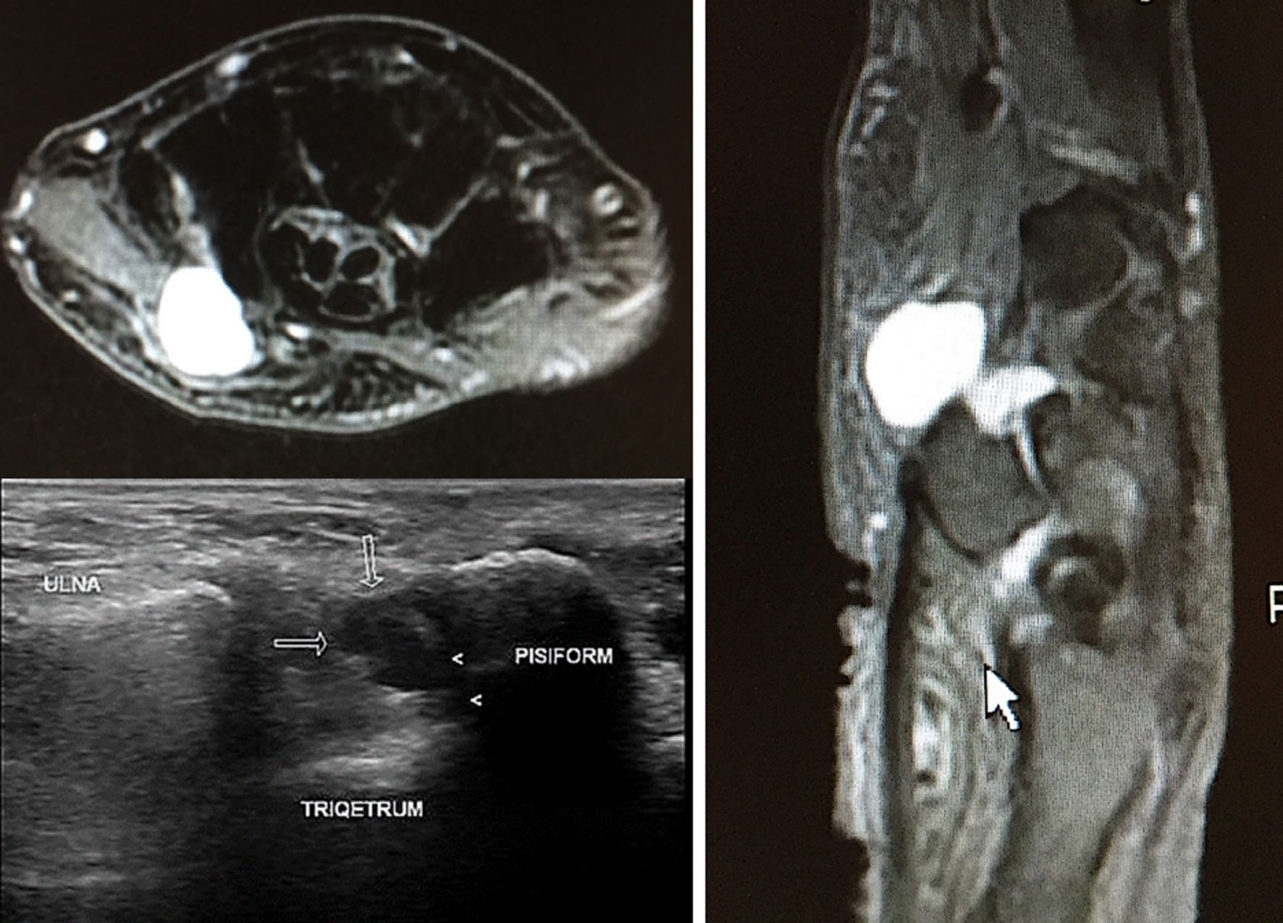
MRI and US of the wrist and hand: huge carpal articular cyst which causes compression of the ulnar motor branch
